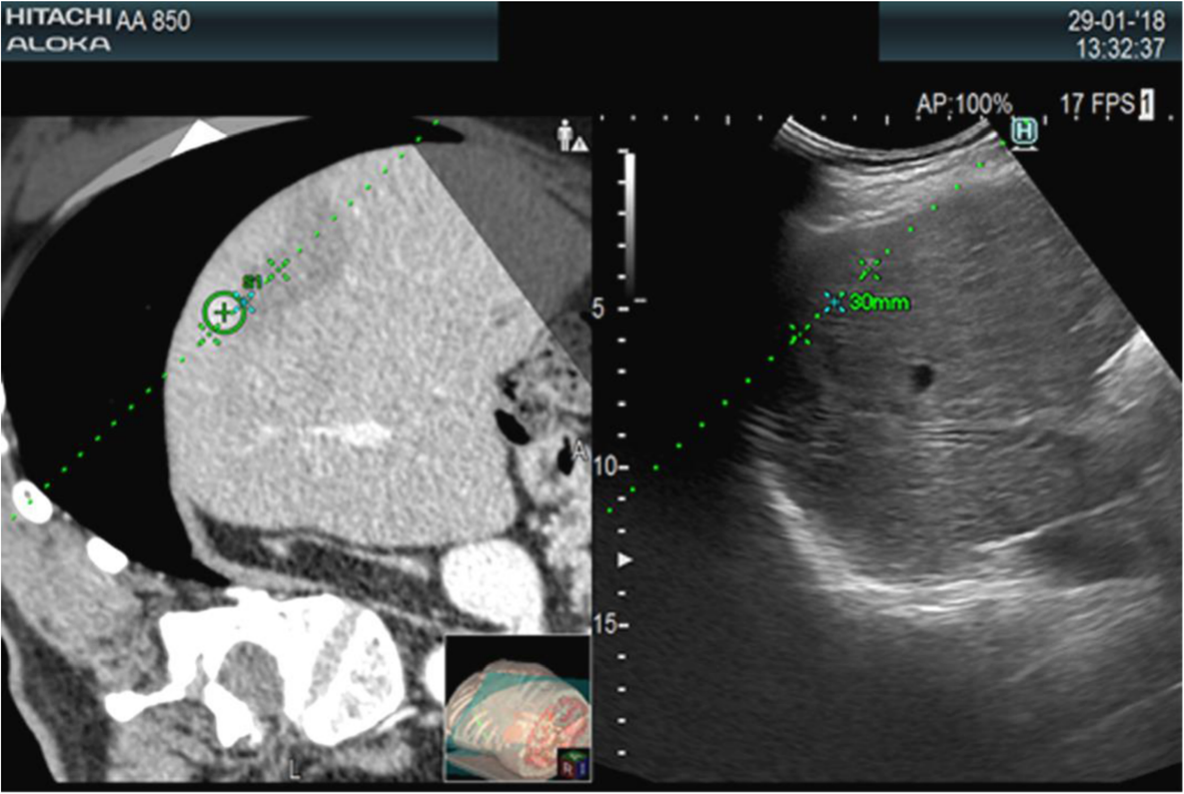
CT-US fusion imaging. Treatment of very small hypervascular nodular recurrence of HCC adjacent to a previously ablated area
